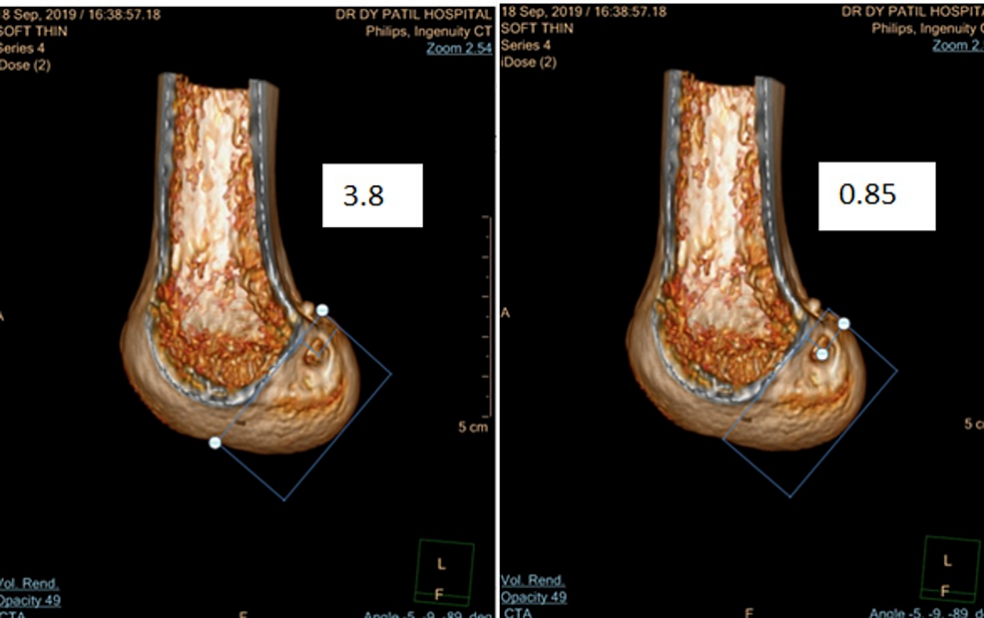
CT image of distal femur showing the tunnel assessment in deep-shallow position (left and right)
